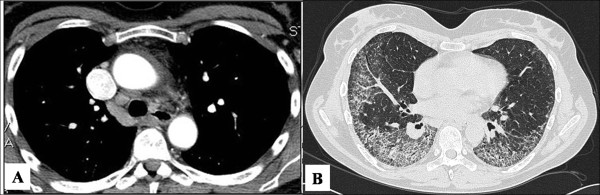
Computed tomography scan of the lung. A: Axial CT scan shows right paratracheal linfoadenopathy B: Axial HRCT scan shows septal interlobular thickening with ground glass opacities at the lower lobes
